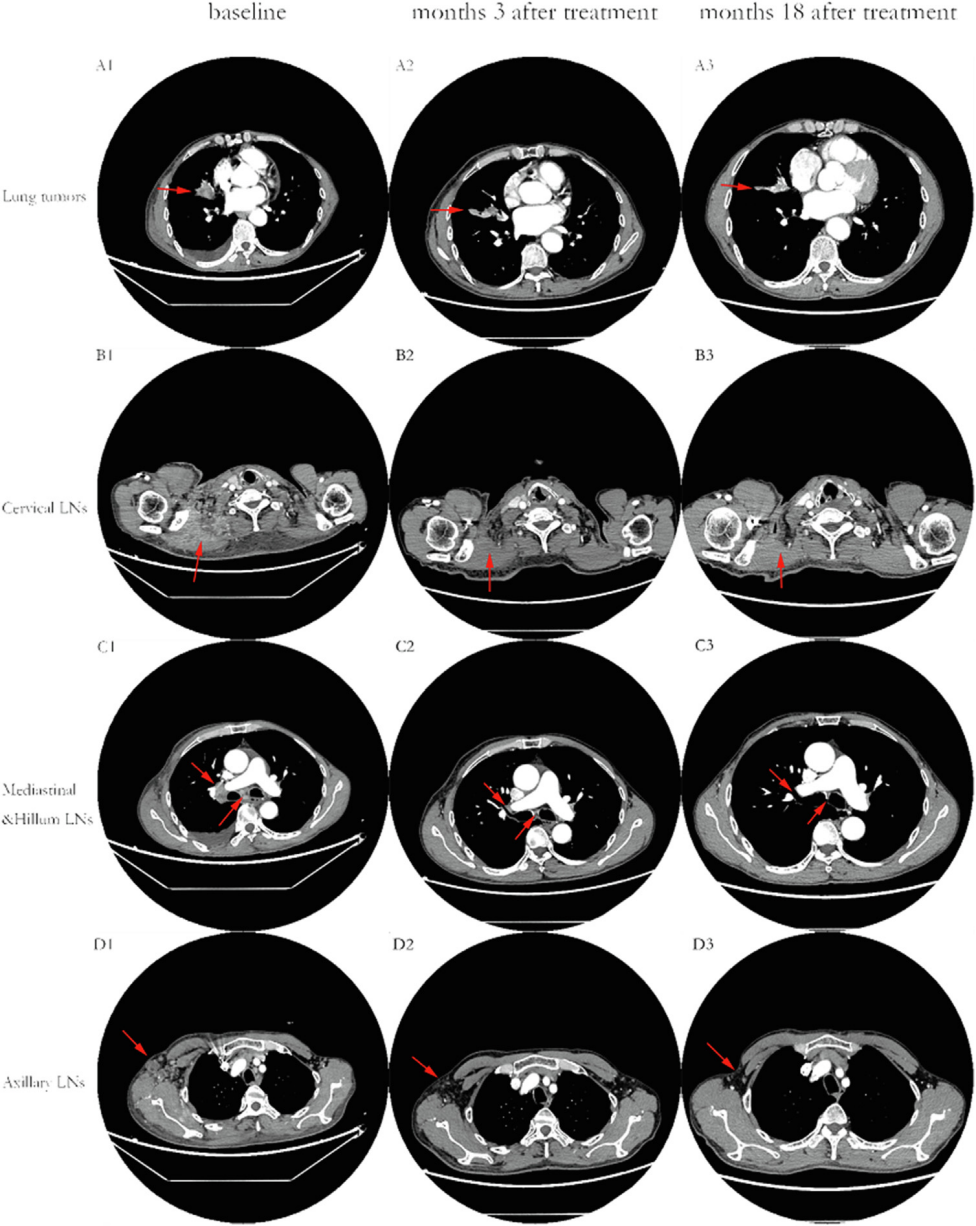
Partial response of CT image findings. (A) The primary foci in the right lung (red arrows) shrank significantly after three months of treatment. (B) The right cervical root lymph nodes (red circles) continued to shrink. (C) The edges of the enlarged lymph nodes in the right pulmonary hilar (large red circles) and mediastinum (small red circles) gradually became clear. (D) The right axillary lymph nodes (red circles) gradually became clearly demarcated from the surrounding tissue. Columns 1, 2, and 3 represent the CT image findings before the start of treatment, three months after treatment, and at the latest follow-up (18 + months), respectively.
